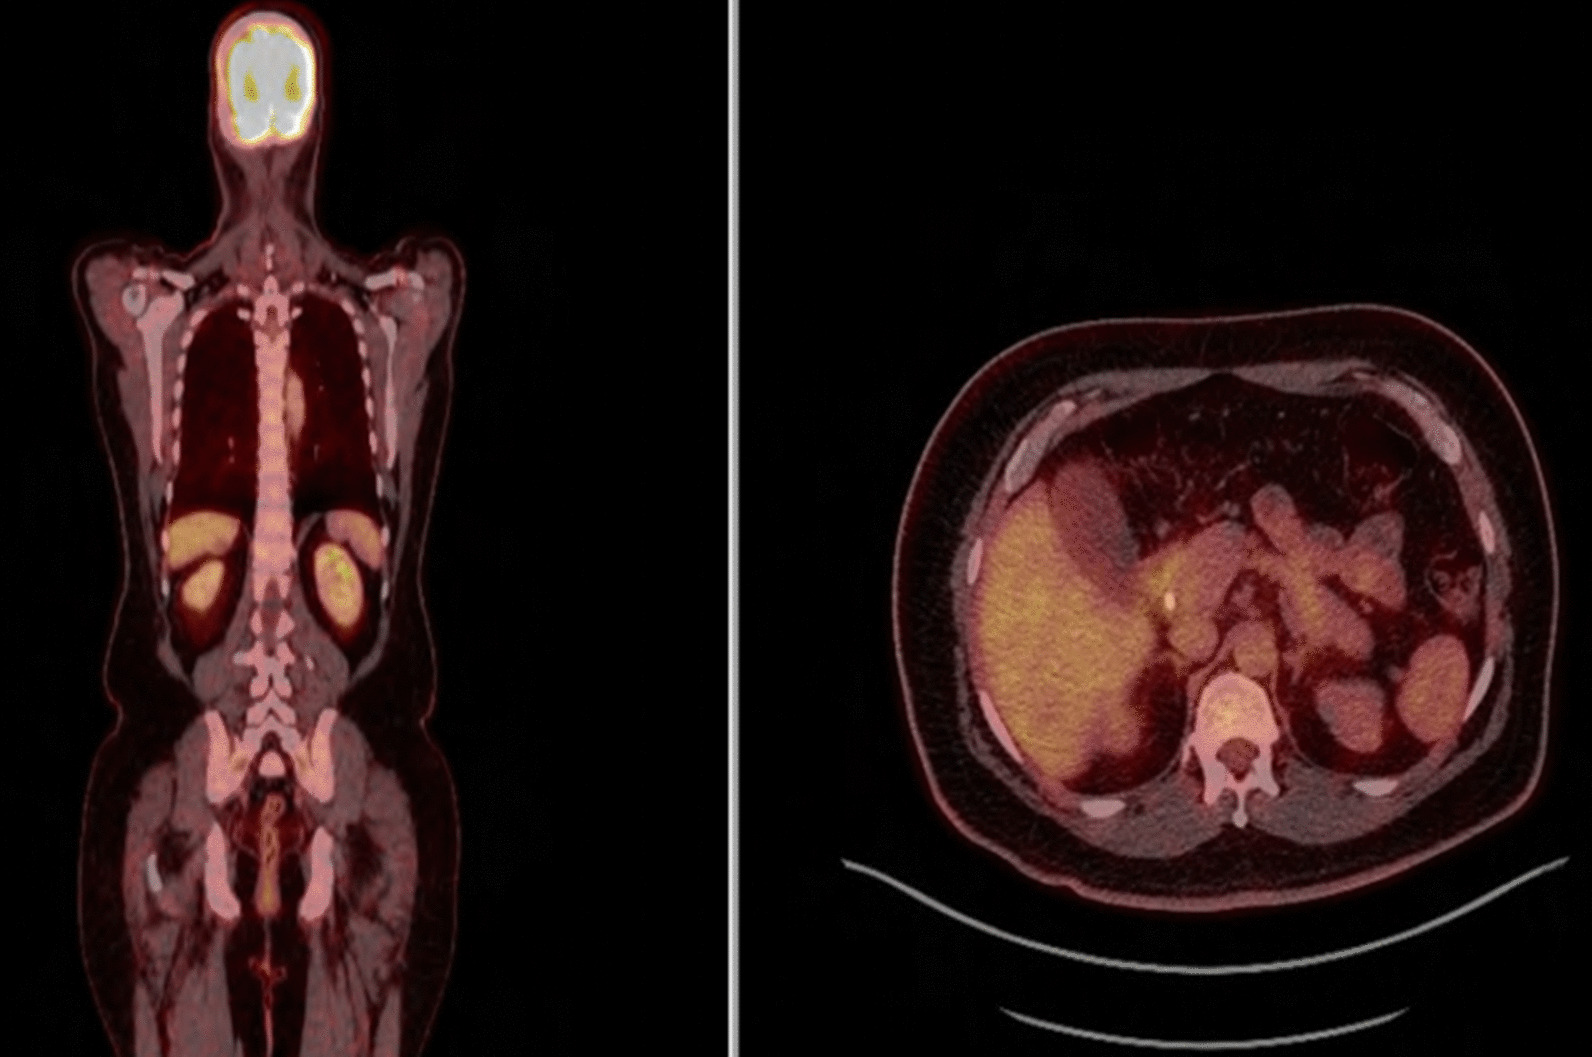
F18 FDG Positron emission tomography-computed tomography images of the coronal liver fusion (left side), Axial fusion (right side). A single hypometabolic hepatic lesion was seen in the right liver lobe (4 × 3 × 3.5 cm^3^). No hypermetabolic lesions were identified in other organs
